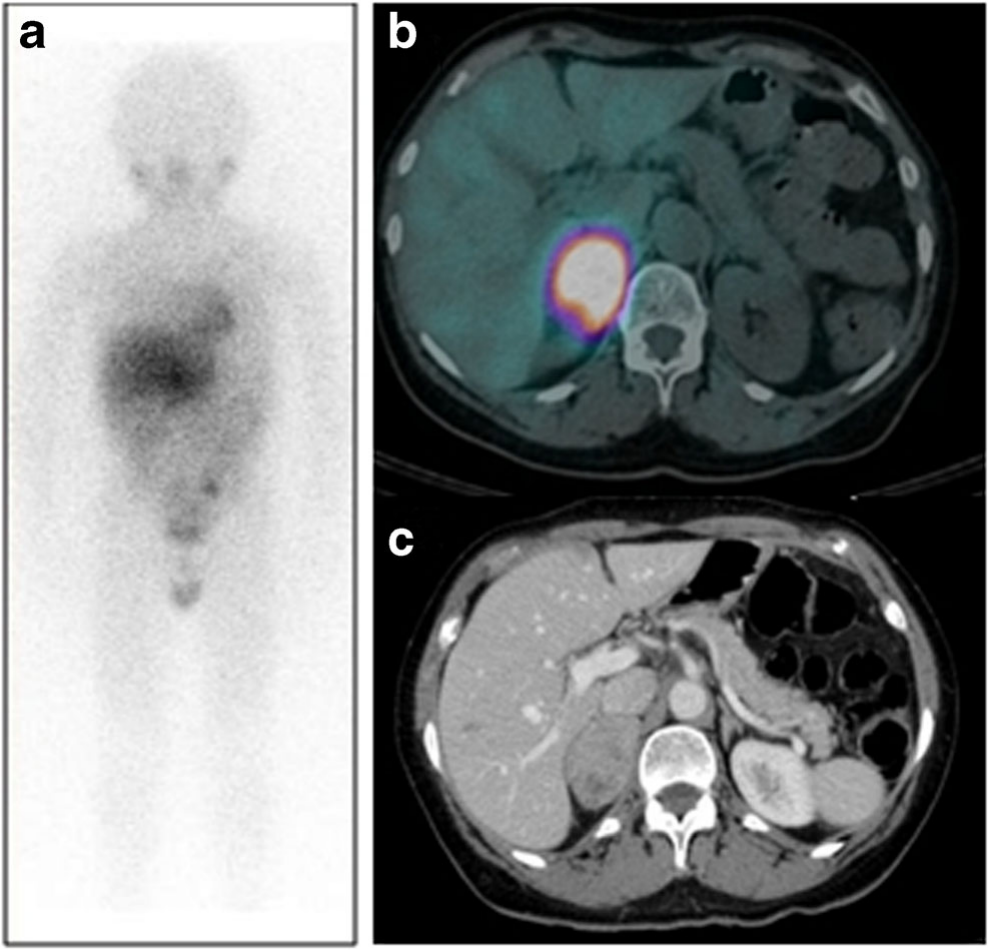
^123^I-mIBG SPECT/CT in neuroblastoma. A 6-year-old child with newly diagnosed neuroblastoma was referred for staging. Planar WB scan (a) shows an area of increased uptake in the right upper abdomen, partially overlapping the liver of unclear location and clinical significance. SPECT/CT (b) localizes this abnormal tracer uptake to the primary lesion in a large mass in the right adrenal seen on CT (C)
